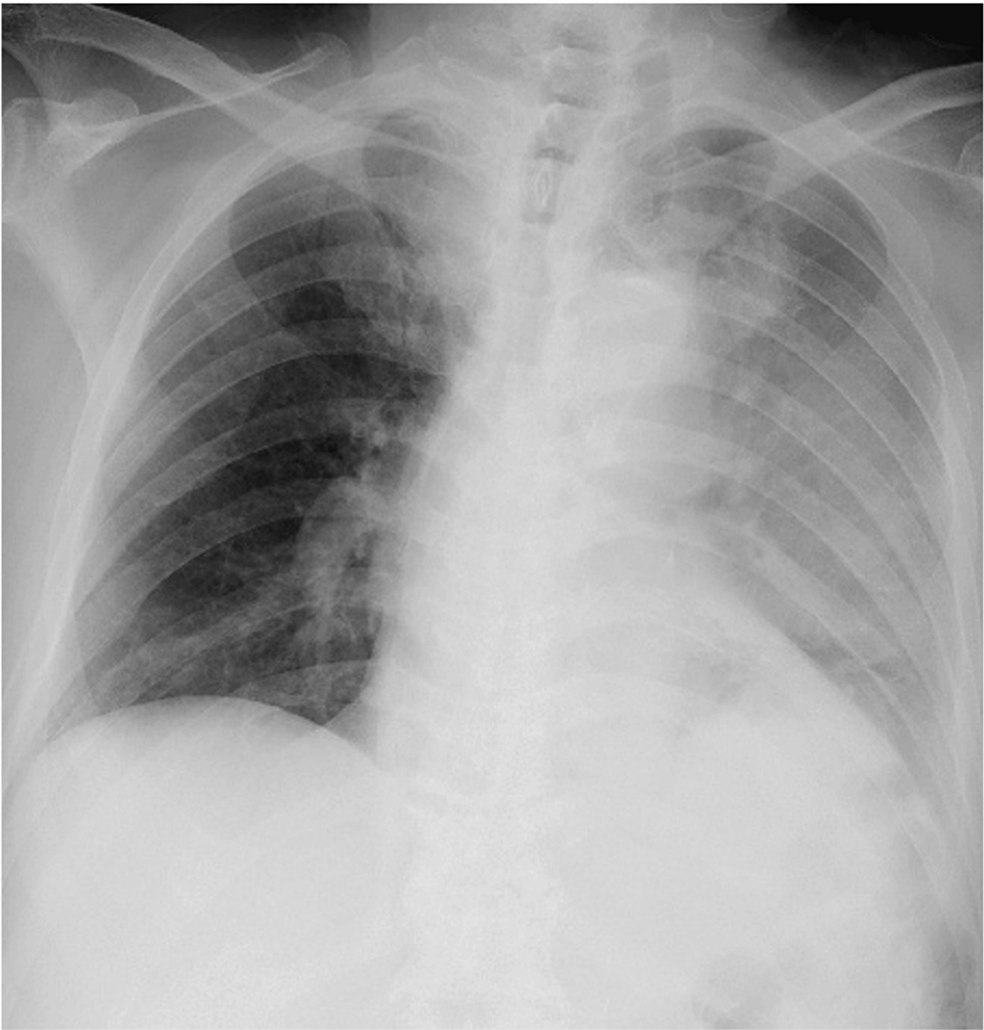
Chest radiography on admission shows an infiltrative shadow in the left lung field.
